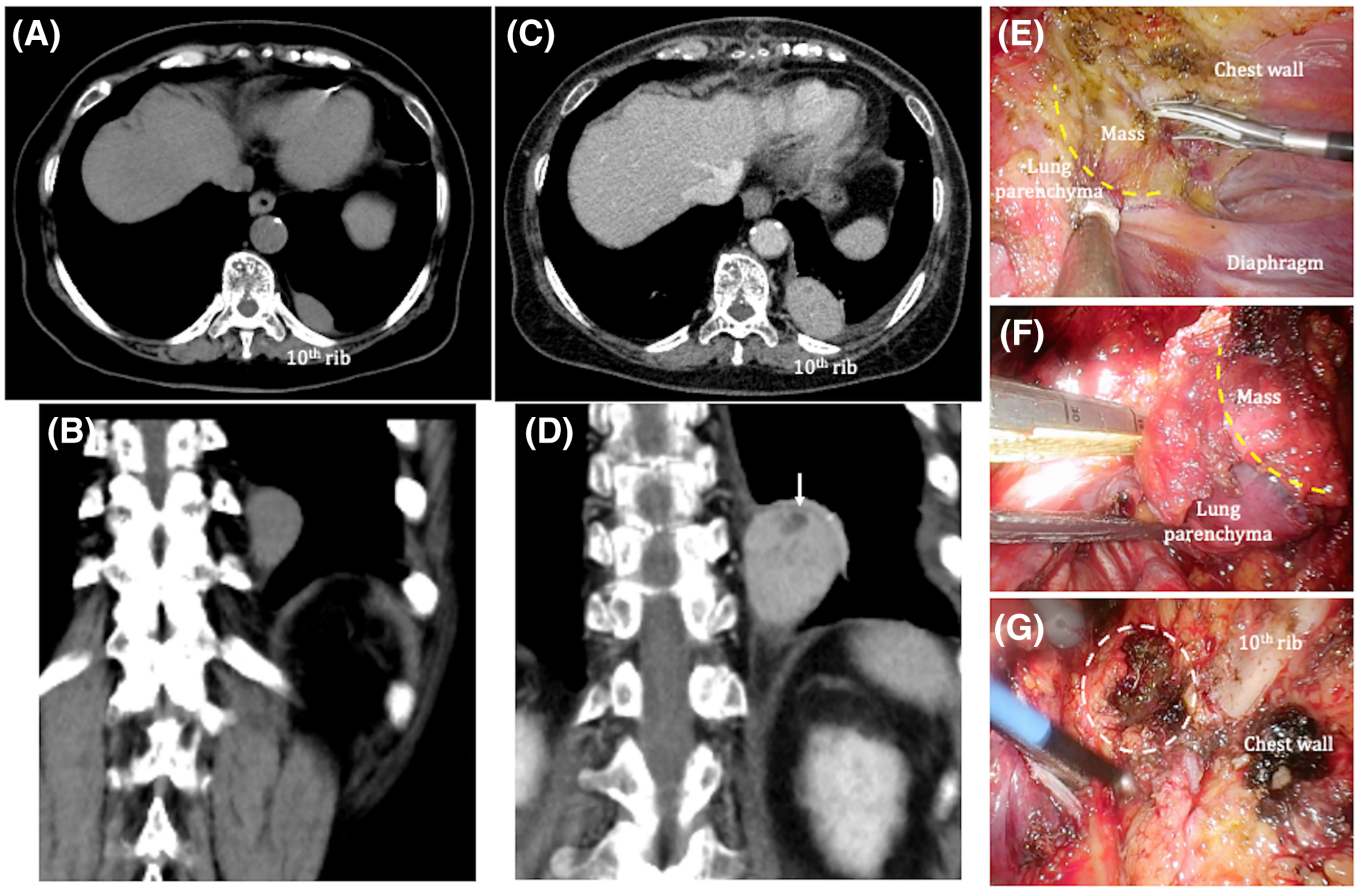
(A, B) Chest computed tomography images taken 6 years prior show a mass measuring 32 × 17 × 32 mm on the left posterior chest wall (near the joint of the head of the 10th rib) with well‐defined margins. (C, D) Contrast‐enhanced chest computed tomography images show a well‐defined mass measuring 41 × 25 × 41 mm on the left posterior chest wall (near the joint of the head of the 10th rib), with some areas of poor contrast enhancement (white arrow). (E, F) The mass is particularly adherent to the adjacent left lower lobe lung parenchyma (yellow dotted lines), necessitating concomitant resection. (G) Intraoperative image after resection shows the area where the mass was located (white dotted circle).
